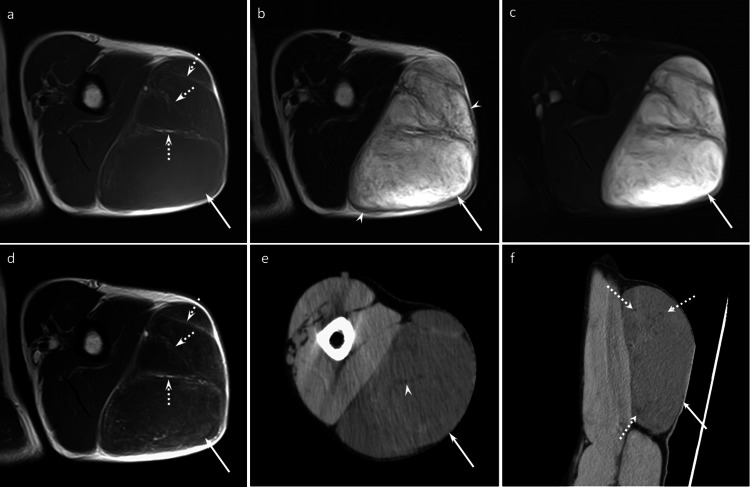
A 53-year-old man with an ASCPLT in the left upper arm Axial T1WI (a), axial T2WI (b), and axial FST2WI (c) show a well-defined subcutaneous non-fatty mass (arrows) with septal/linear fatty components (dotted arrows) and a capsule (arrowheads). The non-fatty components exhibit marked hyperintensity on T2WI. Axial CET1WI (d) shows septal/linear enhancement. Axial CT (e) and coronal CT (f) display a well-defined subcutaneous non-fatty mass (arrow) with a septal/linear fatty area (arrowhead). The imaging findings are consistent with myxoid liposarcoma pattern. T1WI: T1-weighted image, T2WI: T2-weighted image, FS: fat-suppressed, CE: contrast-enhanced.
